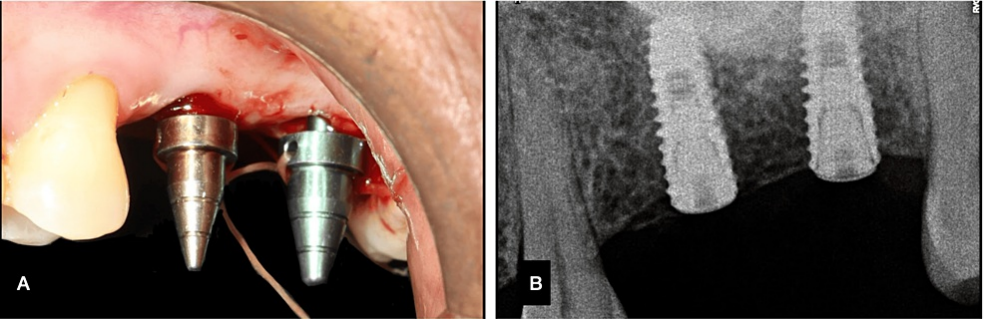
Case 1. A) Clinical PID verification, B) implant placement radiograph.
